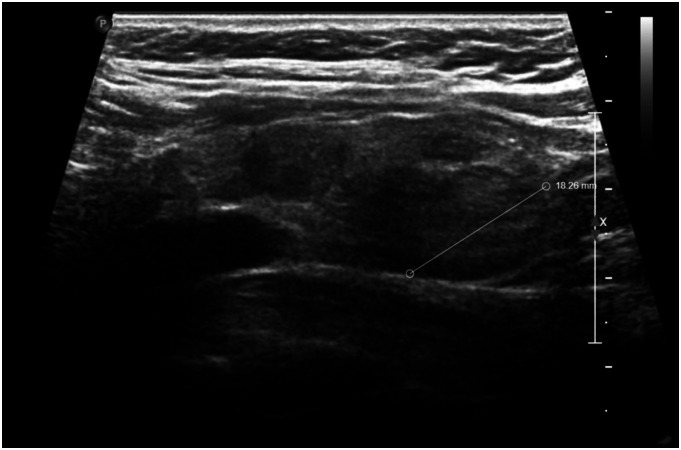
Thyroid ultrasound shows multiple thyroid nodules.
